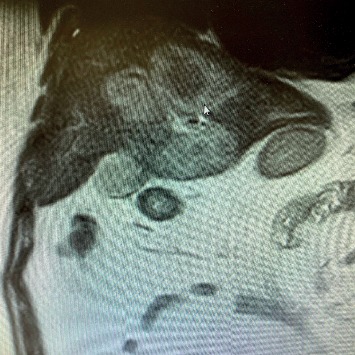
MRI abdomen showing T1 hypointense and T2 heterogeneous hyperintense thin-walled peripheral rim enhancing and intercommunicating cystic mass measuring predominately involving segments IV a, IV b, and I.
